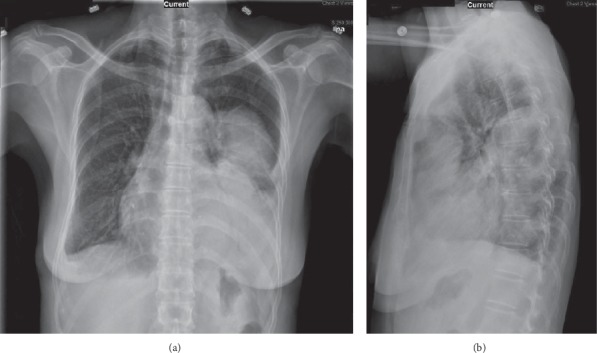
Chest X-ray images of the confluent opacity at the left lower hemithorax. Frontal view (a) and lateral view (b).
